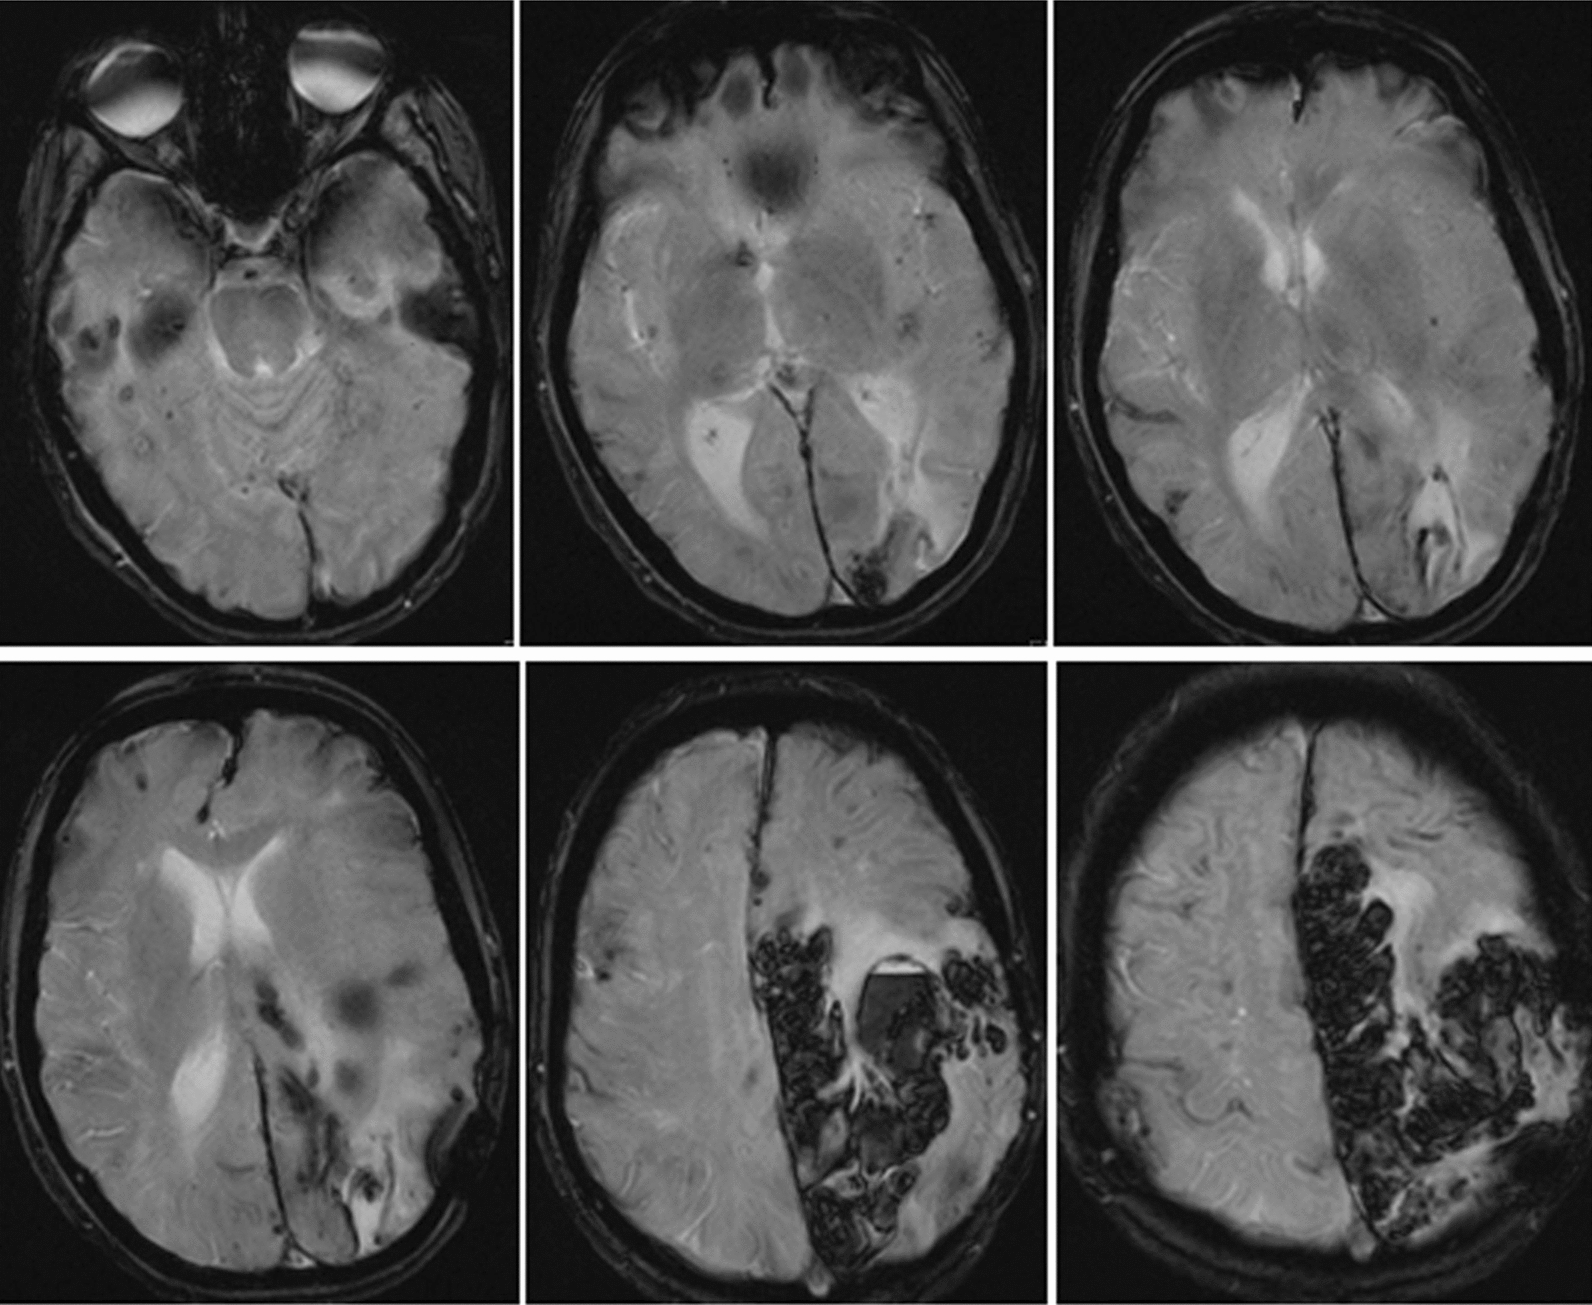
MRI T2* sequences showing a wide left fronto-temporo-parietal haematoma with several bilateral microbleeds
